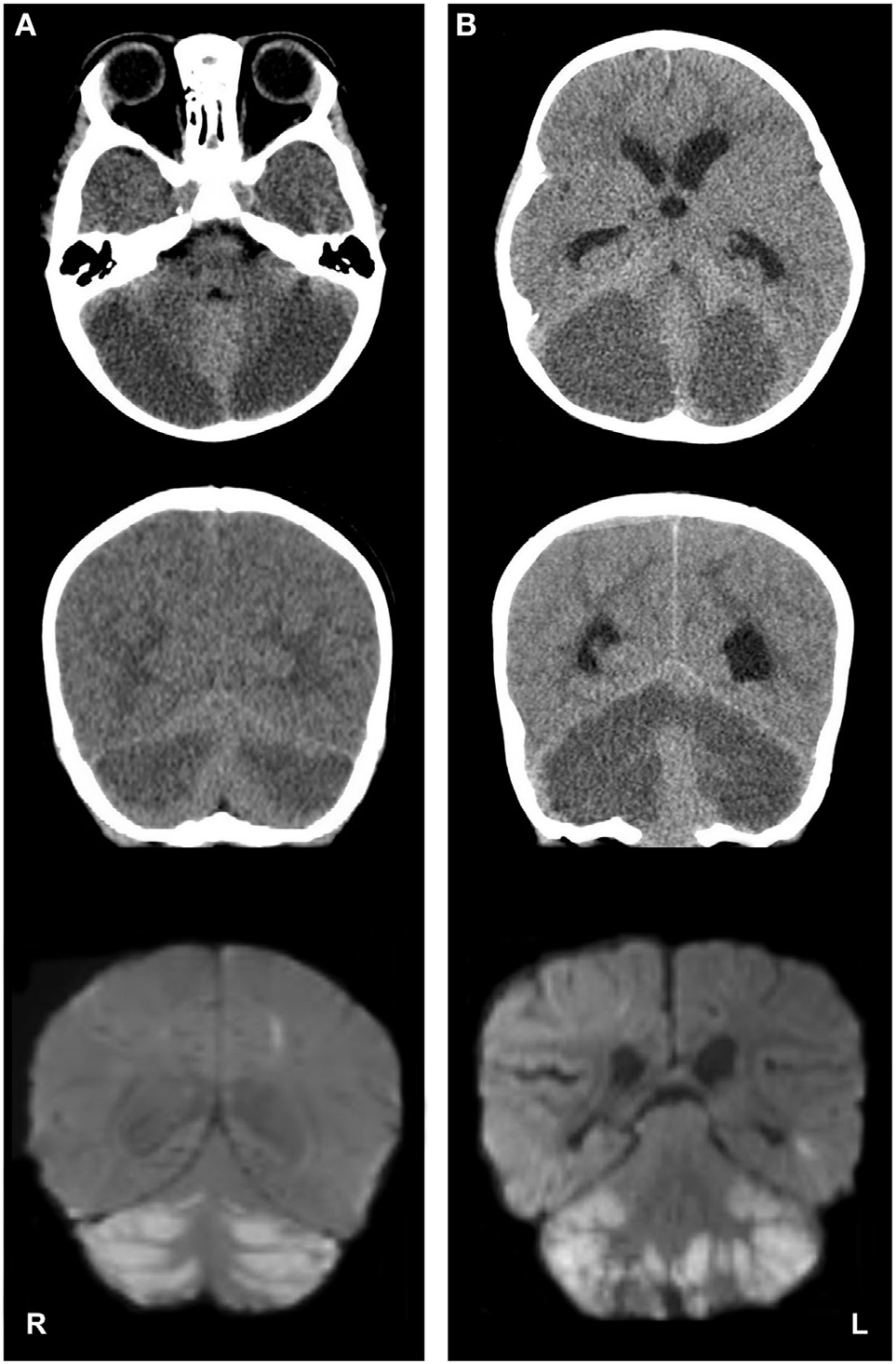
Imaging studies depicting cerebellar edema and restricted water diffusion after accidental opioid overdose in two infants. Panel (A), case 1; panel (B), case 2. Top row: axial computed tomography (CT) images show severe, bilateral cerebellar hypoattenuation, with ventral displacement of the cerebellar vermis and compression of the fourth ventricles. Middle row: coronal CT images demonstrate bilateral cerebellar hypoattenuation and upward displacement of the tectal plate. Bottom row: coronal magnetic resonance diffusion-weighted imaging (DWI) depicts bilateral, symmetric cerebellar diffusion restriction [note: DWI images on panel (B) are postoperative].
